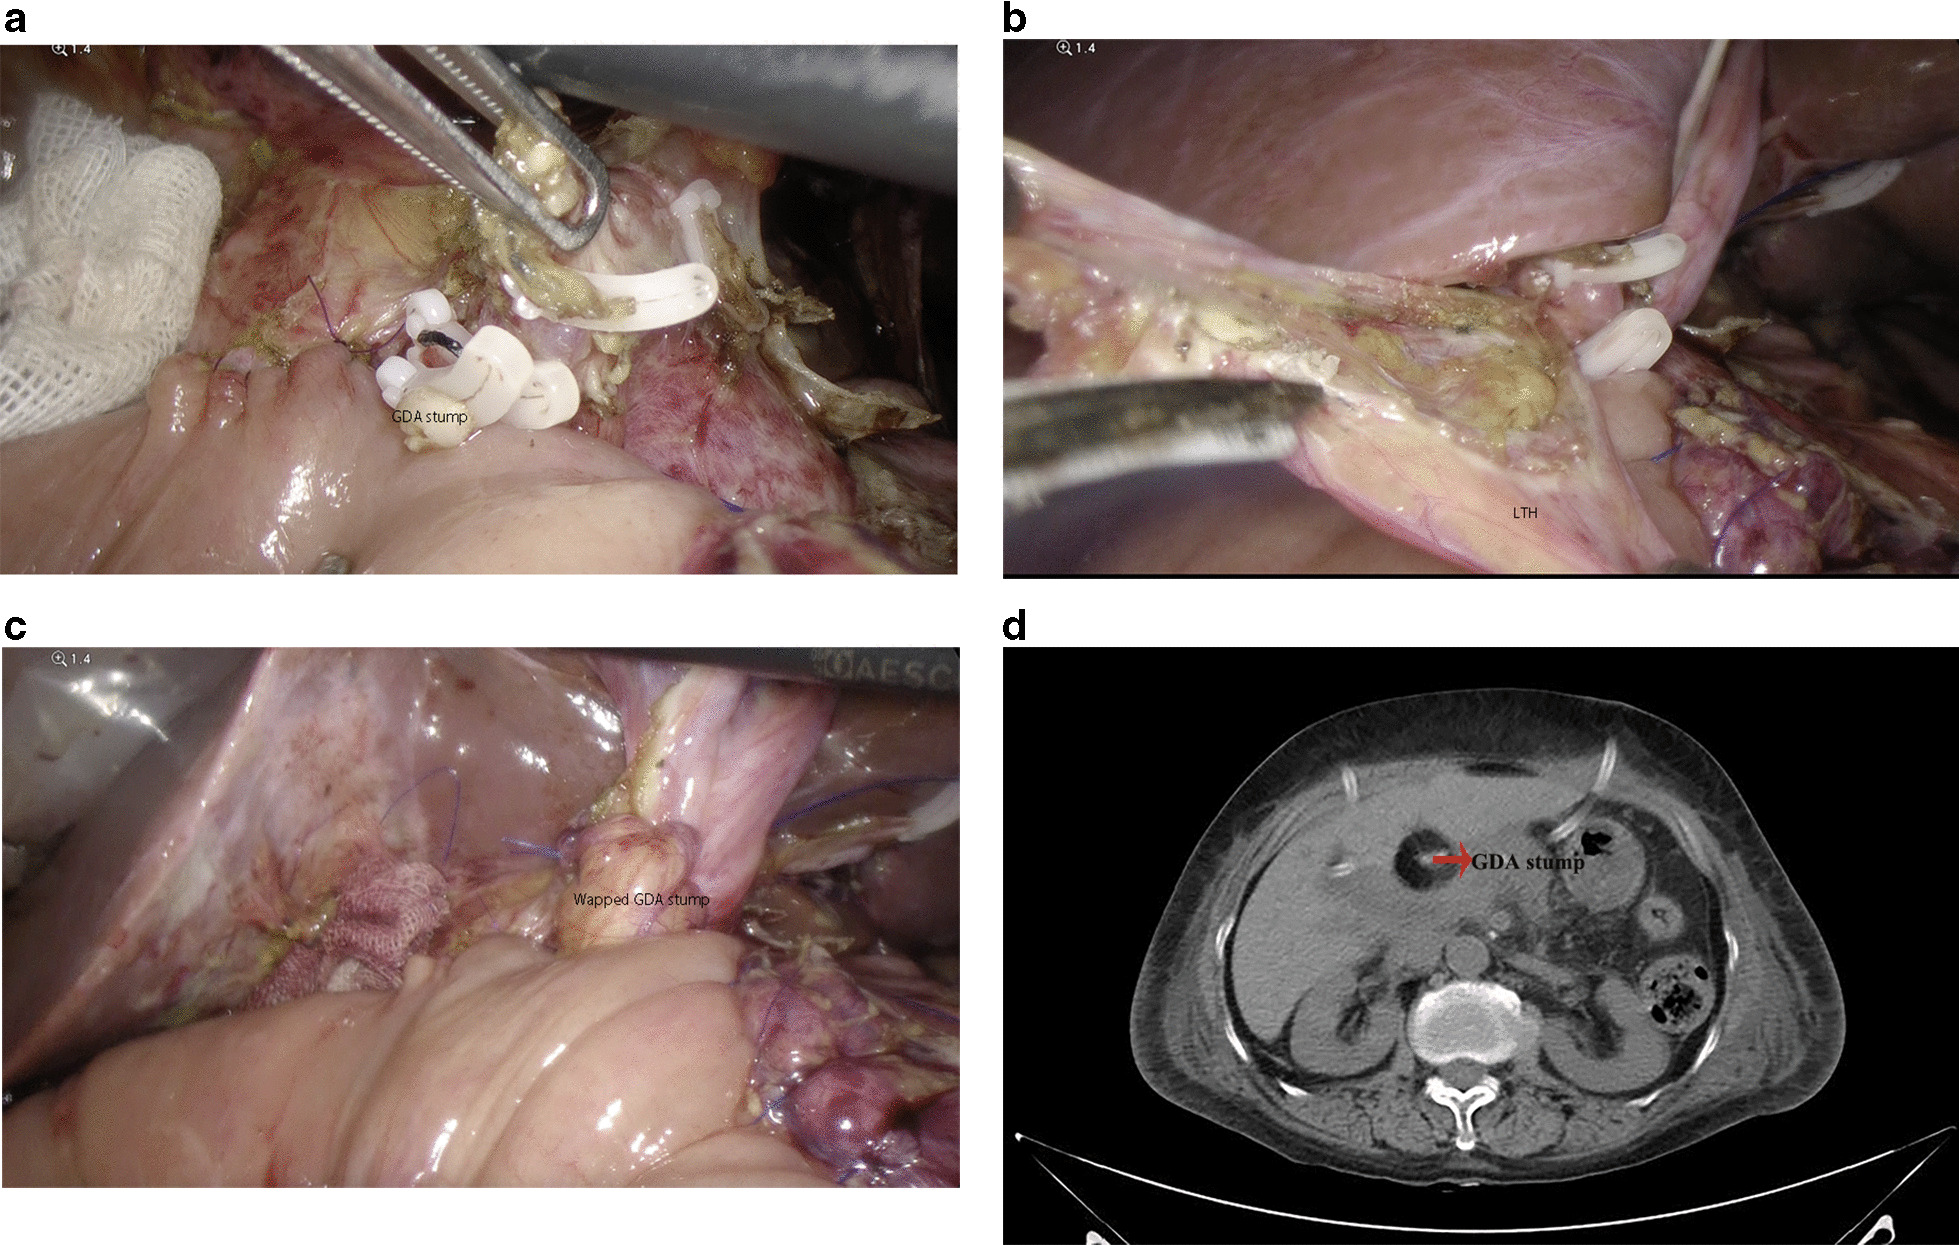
a The LTH is detached from the abdominal wall from the umbilicus to the liver. b After the anastomosis, the LTH is divided, extended used as a vascular pedicle. c The LTH is used to cover the GDA stump. d Postoperative CT scan showing the wrapped GDA stump after a LPD procedure
